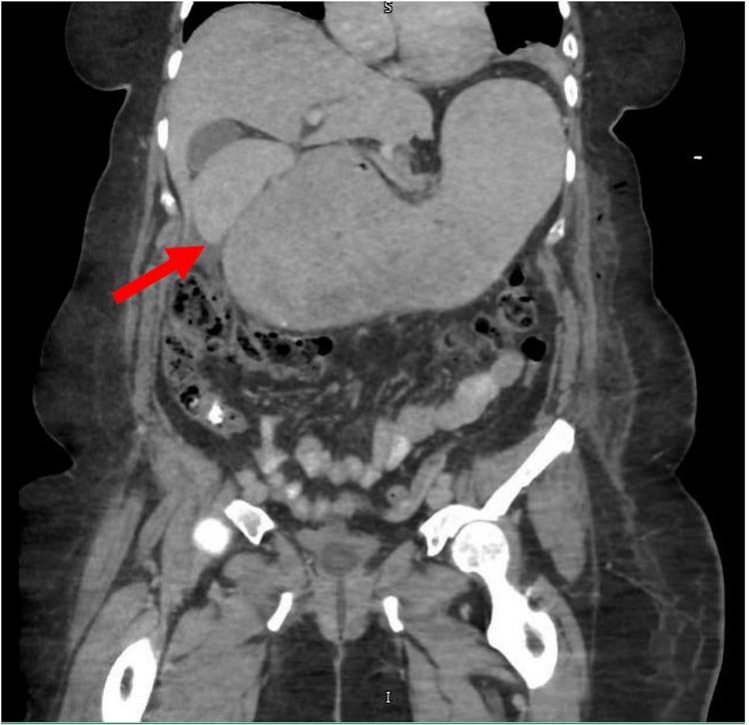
CT of the abdomen and pelvis (coronal slice) showing gastric distention and the spleen positioned in the right upper quadrant.
